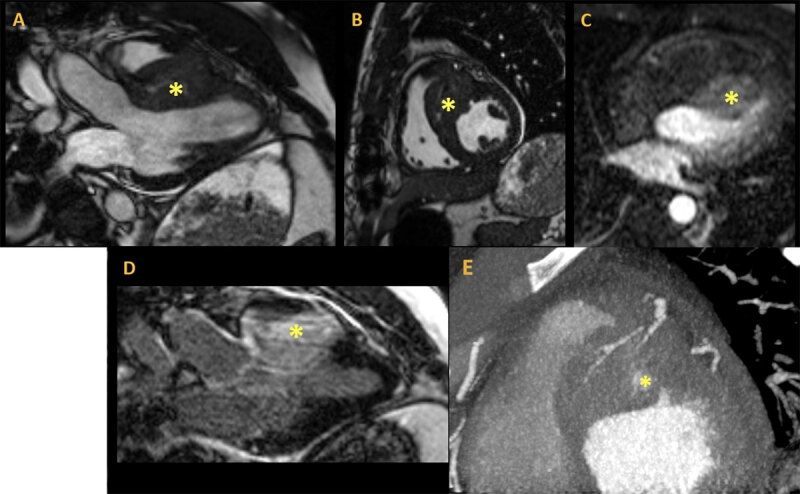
Preoperative cardiac imaging. Magnetic resonance imaging using steady state free precession cine sequence: (A) 3-chamber view, (B) short axis at the mid-level, (C), first pass perfusion, and (D) delayed enhancement after gadolinium showing a large right ventricular (RV) mass measuring 4.9 × 3.7 cm invading the RV free wall, with (E) intense first pass perfusion and significant late gadolinium enhancement cardiac computed tomography demonstrating short axis basal anteroseptal mass protruding into the RV outflow tract with central contrast enhancement and multiple septal perforator feeding vessels.
